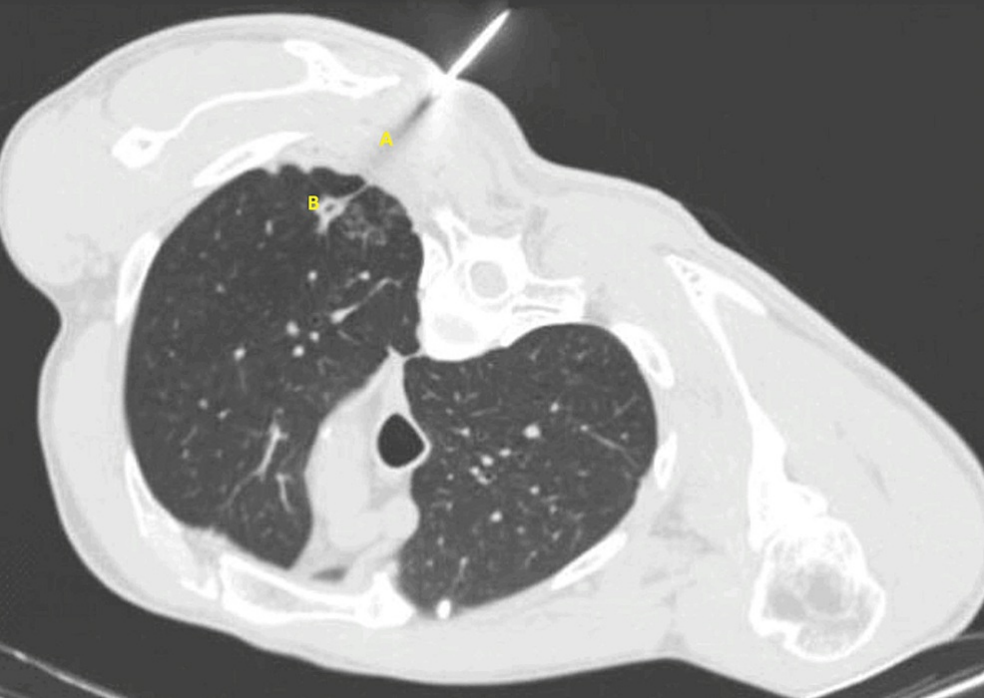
Transthoracic needle biopsy axial CT scan image showing posterior transthoracic needle biopsy with proper placement of introducer needle at edge of left upper lobe nodule (B) and the resulting pathway (A)
